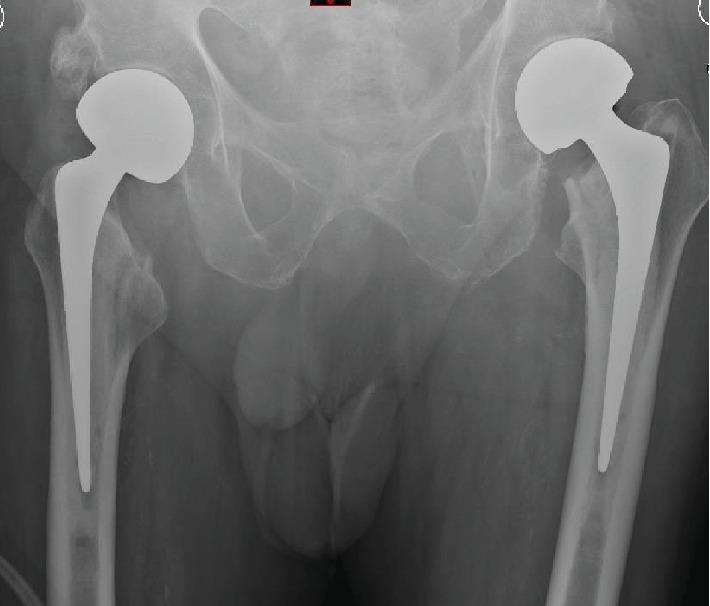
Postoperative radiograph showing bilateral cemented Exeter hemiarthroplasties.
